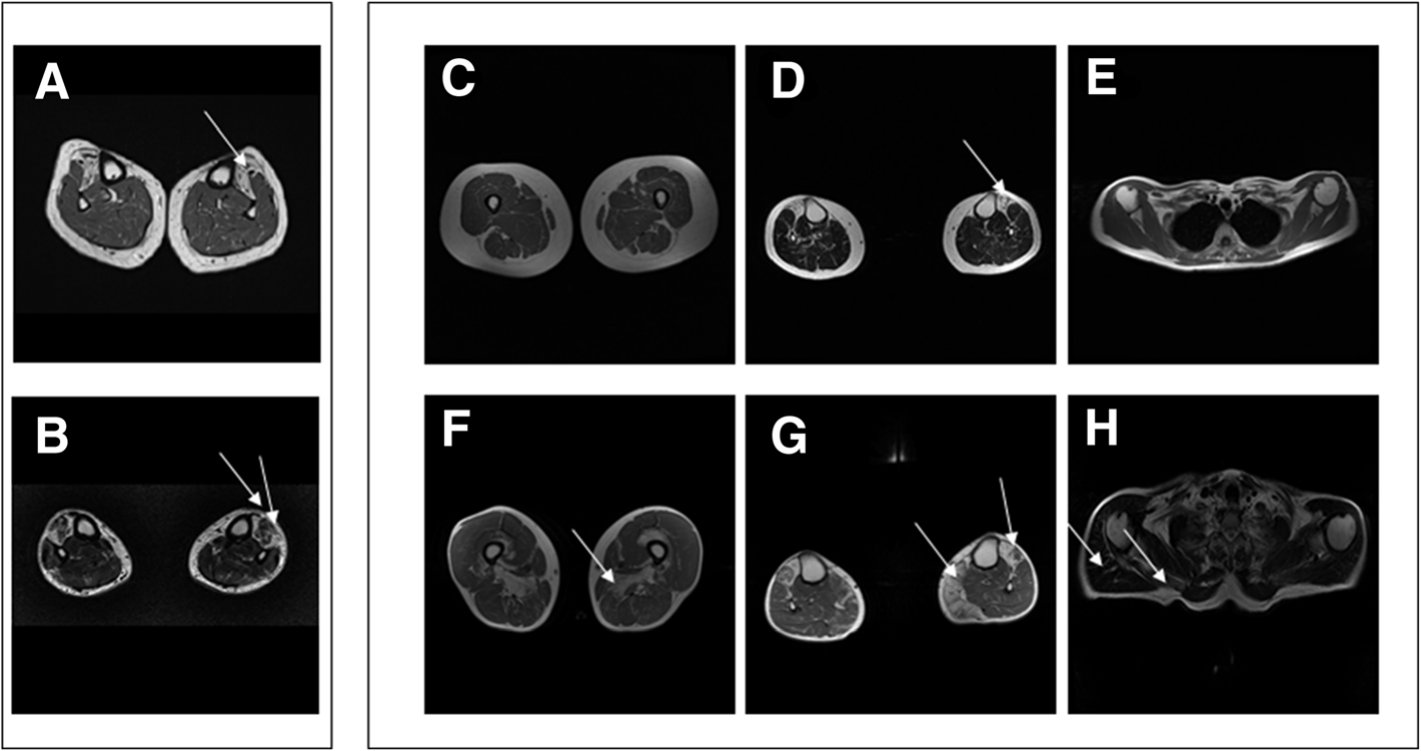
Myo-imaging in family members. Muscle MRI findings at calf level in III.4 (a) and III.2 (b) were obtained using conventional T1-weighted spin-echo transverse images. Both scans show the selective involvement of the tibialis anterior and, in III.2, of the extensor digitorium longus with sparing of gastrocnemii and soleus. Follow-up muscle MRI after 3 years in III.4 (c–e) and III.2 (f–h) obtained using conventional T1-weighted spin-echo transverse images at thigh (c, f), calf (d, g) and upper girdle (e–h) level. The findings in III.4 are almost stable whereas in III.2 it is possible to observe the additional involvement of the adductor magnus and vastus intermedius at thigh level (f) and of the medial gastrocnemius at calf level (g). The scans of the upper girdle reveal involvement of the deltoid and sternocleidomastoid muscles
